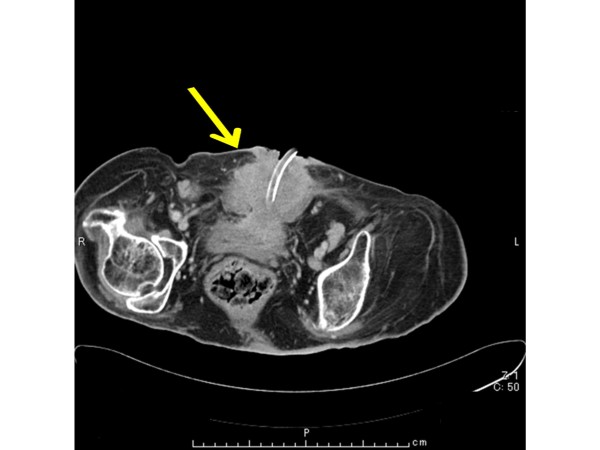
An enhanced abdominal CT showed a mass (72 mm × 63 mm) surrounding suprapubic.
